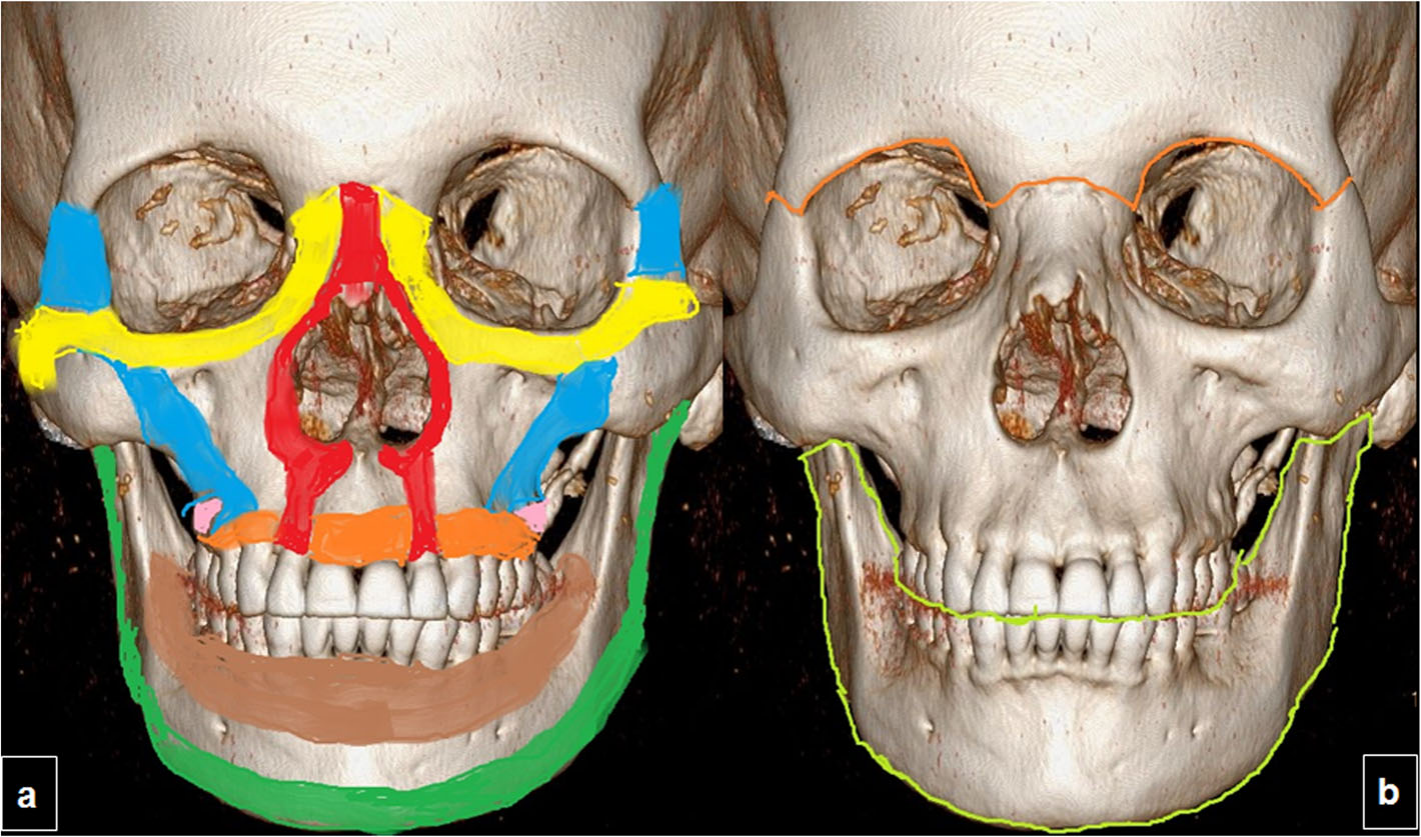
Facial anatomy. a System of facial struts/buttresses. Three-dimensional CT images of an adult skull in frontal view with color overlays. The horizontal buttresses are the upper transverse maxillary (yellow), lower transverse maxillary (orange), upper transverse mandibular (brown), and lower transverse mandibular (green) buttresses. The vertical buttresses are the medial maxillary (red), lateral maxillary (blue), posterior maxillary (pink), and posterior mandibular (green) buttresses. b System of facial partitions. Three-dimensional CT image of an adult skull with color overlays shows partition of facial anatomy into upper (outlined in orange), middle, and lower (outlined in green) thirds, the system used by otolaryngologists to describe locations of fracture
